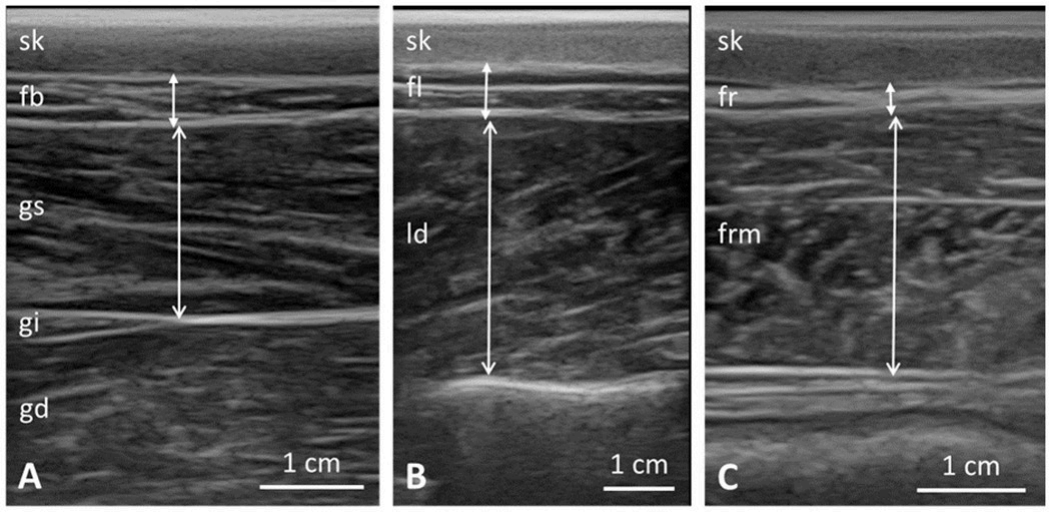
Photographs showing the three locations of ultrasound measurements. Measurements were made at the buttock at the point of convergence of the facia of the superficial, intermediate, and deep gluteus muscles (A); at the spine of the 4th lumbar vertebra (B); and between the 12th and 13th ribs, at the tip of the ribs with the probe placed vertically (C). The bar represents 1 cm in all photographs. Double white arrows and double white open arrows represent the locations where measurements were performed for fat and muscles, respectively. sk: skin, fb: fat thickness at the buttock, gs: gluteus superior thickness, gi: gluteus intermediate, gd: gluteus deep, fl: fat thickness at the lumbar region, ld: longissimus dorsus thickness, fr: fat thickness at the flat rib region, frm: flat rib muscle thickness.
